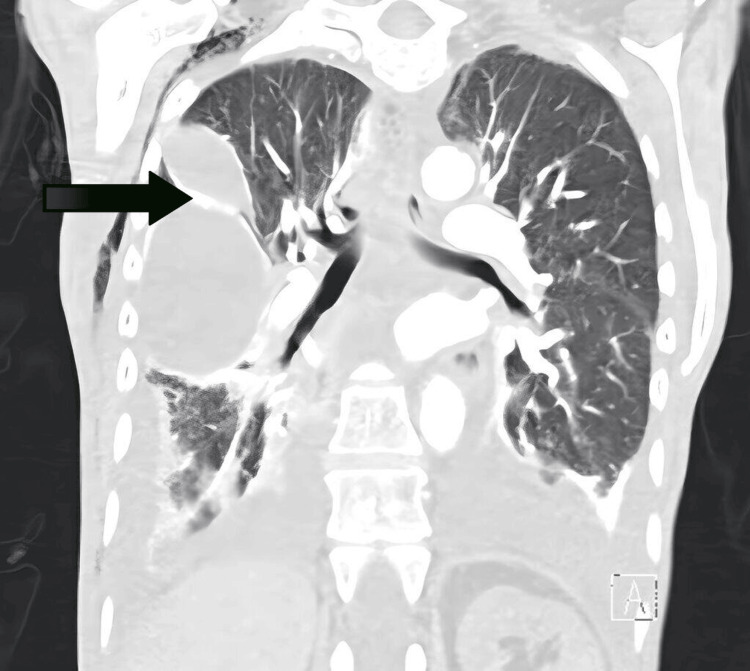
CT showing complex large loculated pleural effusion in the right side (arrow) CT: computed tomography
